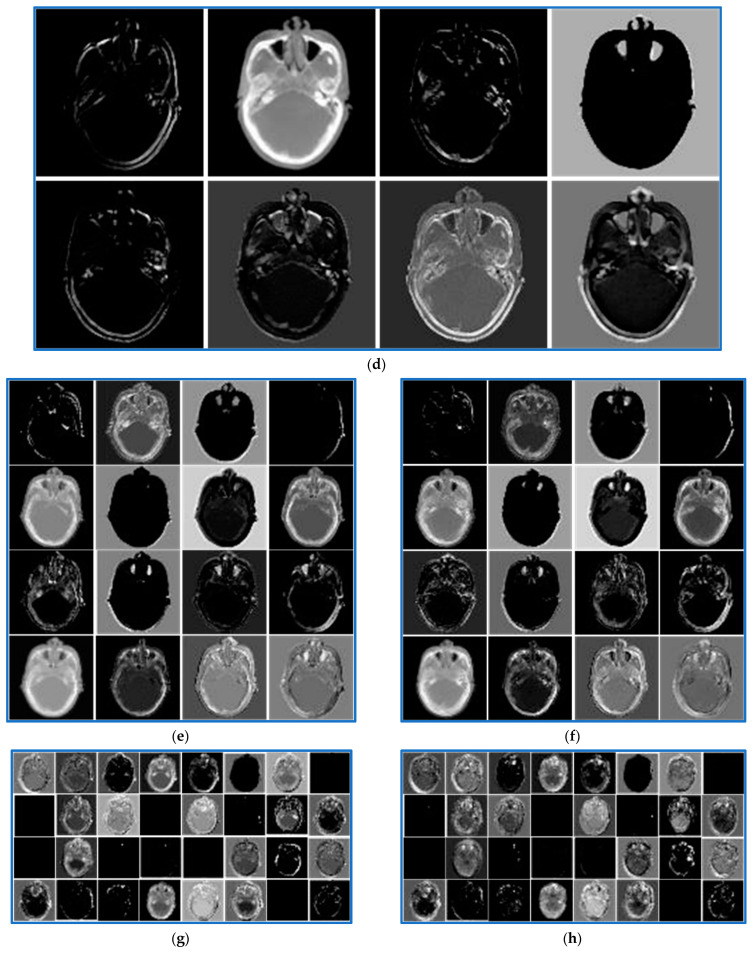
Slice examples of CT and CBCT feature maps at different scales. (a,b) The original image slices of CT and CBCT, respectively. (c–h) The feature maps at different resolution levels produced by the first three layers, with the resolution halved after each layer. Specifically, (c,e,g) are feature maps of CT images, while (d,f,h) are feature maps of CBCT images.
